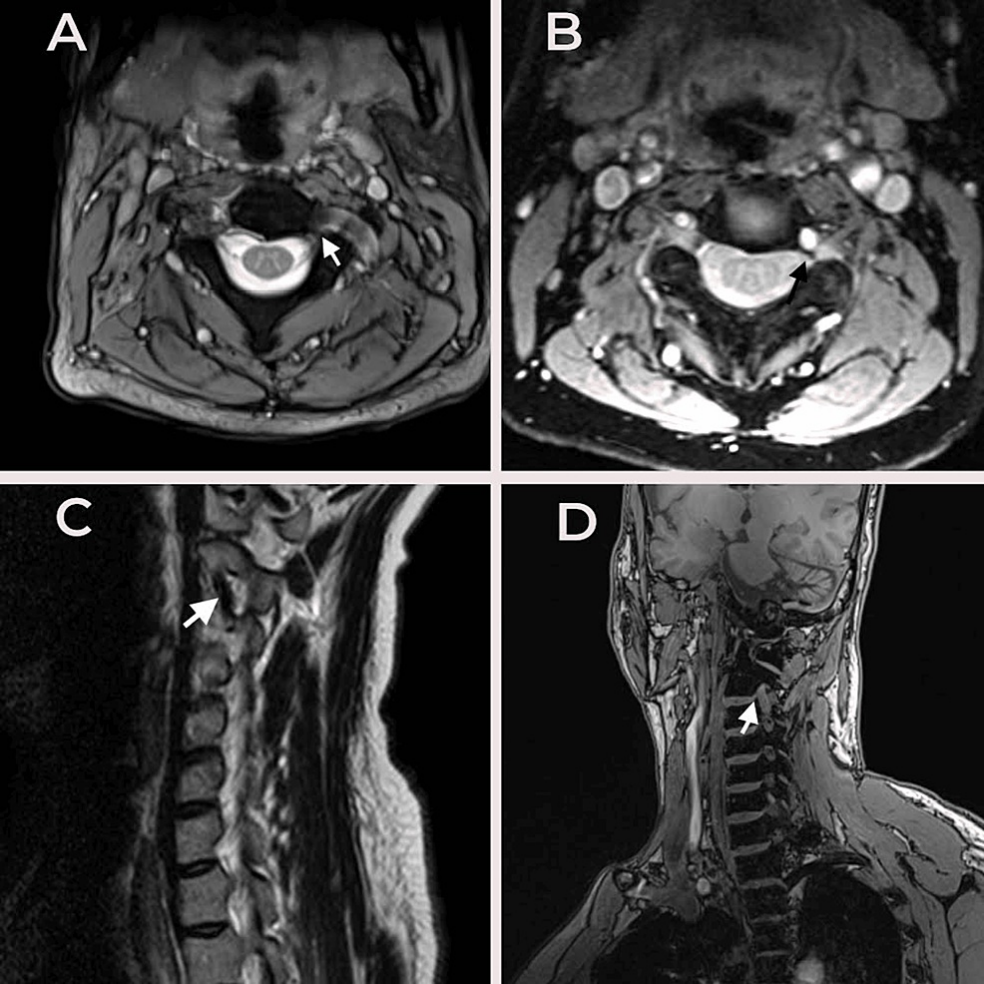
(A) T2 MERGE, (B) MRA, (C) T2 sagittal MRI, and (D) T1 VIBE MR The arrows point to the abnormal left vertebral artery course within the foramen, compressing the C3 root. MRA, magnetic resonance angiography; MRI, magnetic resonance imaging
